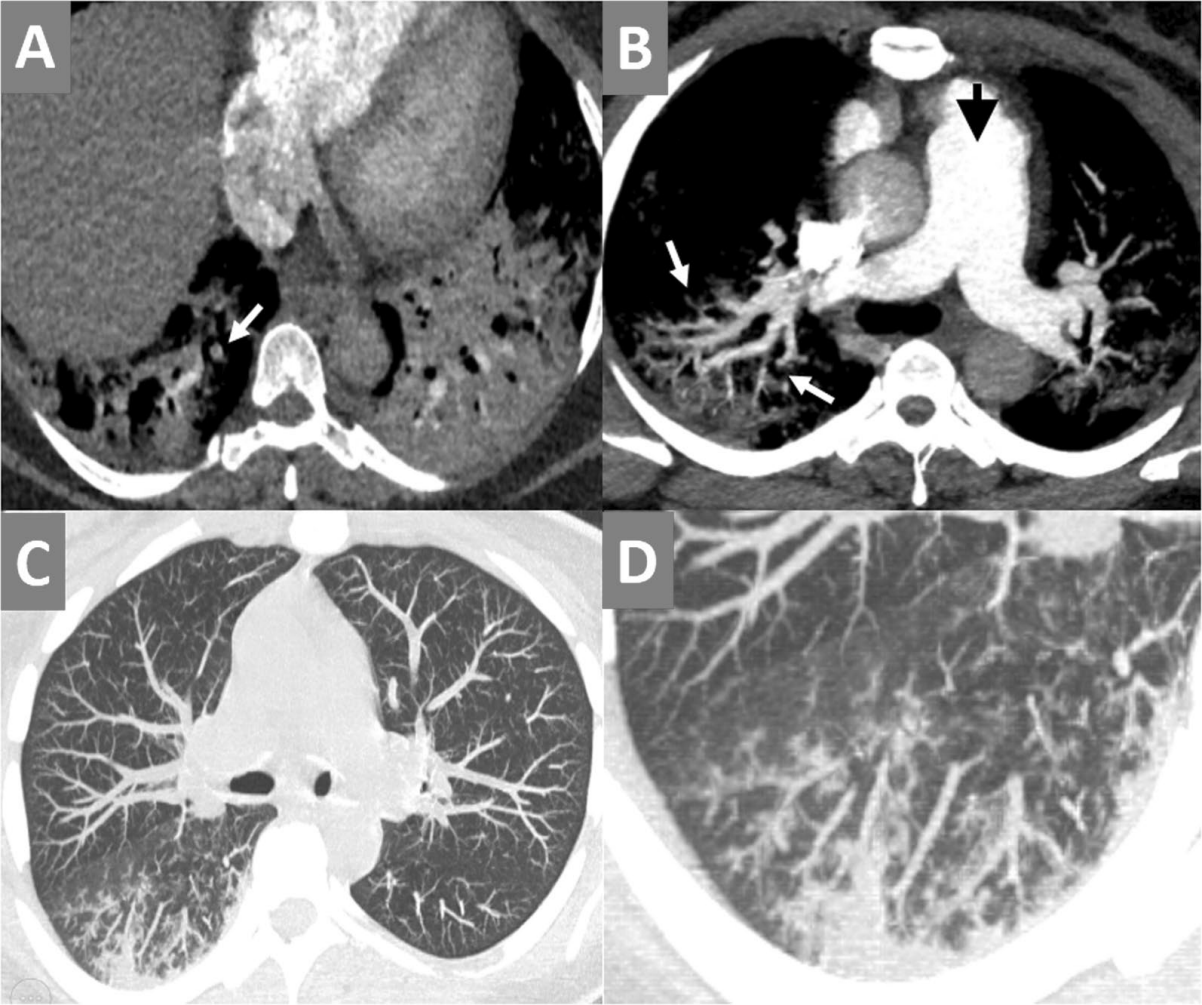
CT-pulmonary angiography (CTPA) examination for a 57-years-old COVID-19 male patient complained of severe chest pain and sudden deterioration of oxygen saturation in the second week of infection. [A] Axial CTPA image showed small contrast filling defect inside one of the right basal pulmonary arterial branches … Acute pulmonary embolism. This is together with bilateral basal consolidative and atelectatic patches. [B] Axial CTPA image showed dilatation of the pulmonary trunk exceeding the caliber of the nearby aorta (black arrow) referring to pulmonary hypertension. This is together with a right basal pulmonary vascular enlargement (white arrows). [C] Corresponding axial CT image with MIP reconstruction (lung window) and [D] zoomed image showing branching beaded nodular pattern continuous with the course of the pulmonary arterial tree … Vascular tree-in-bud sign (an indirect sign of pulmonary vasculopathy)
